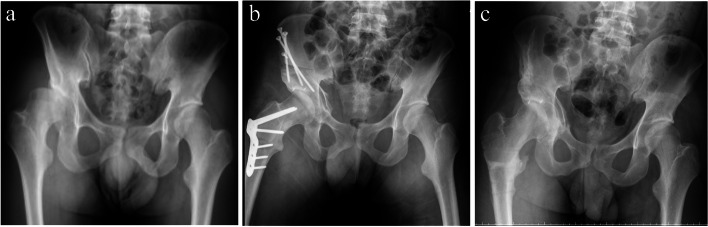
A 21-year-old male, diplegia. Pre-op GMFCS was II. Post-op GMFCS was II. a Pelvic radiography before surgery; b immediately after surgery of PAO + VDRO as well as labrum repair; c two years after surgery
